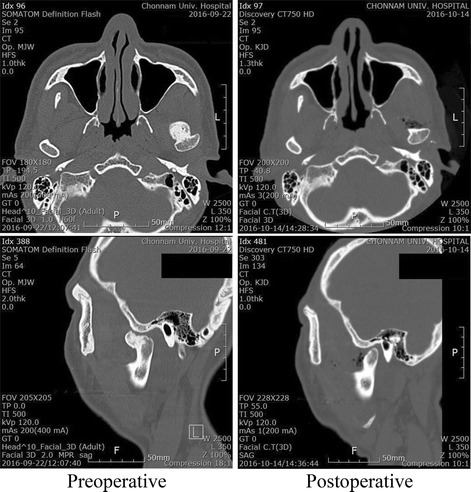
Preoperative CT scan (*left*) and postoperative CT scan (*right*)
